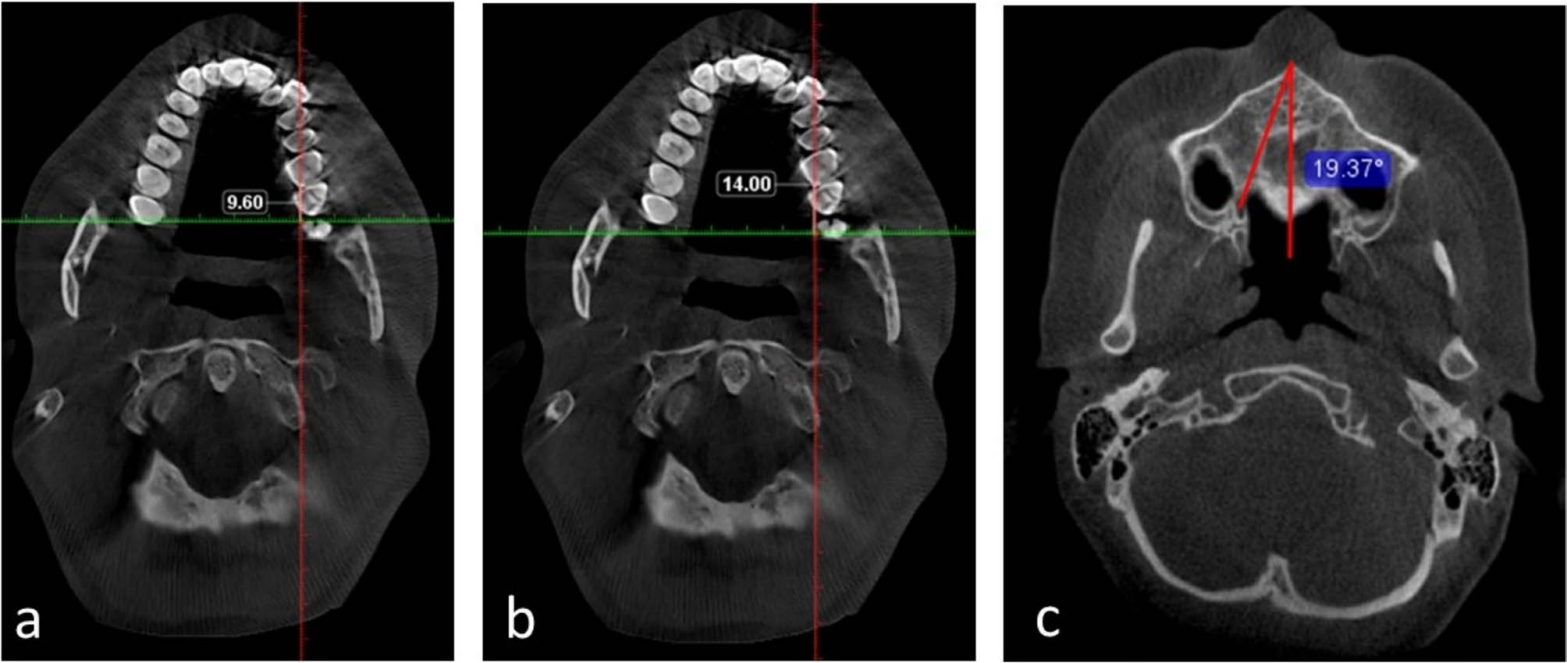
a Axial CBCT reformatted image showing the distance between GPF anterior border and the distal surface of 1 st molar (white line). b Axial CBCT reformatted image showing the distance between GPF posterior border and the distal surface of 1 st molar (white line). c Axial CBCT reformatted image showing MMS–IF–GPF angle (red curved line)The distance between the posterior margin of GPF and the distal surface of 1 st molar in mm [GPF(P)−1st molar distance] (Fig. 3b).
